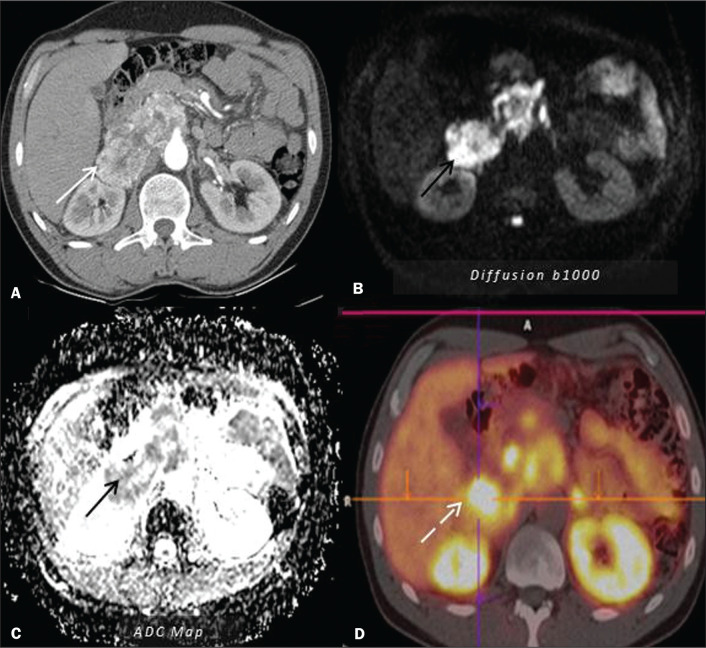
Paraganglioma. CT (A) and MRI (B,C), showing an expansile, solid
